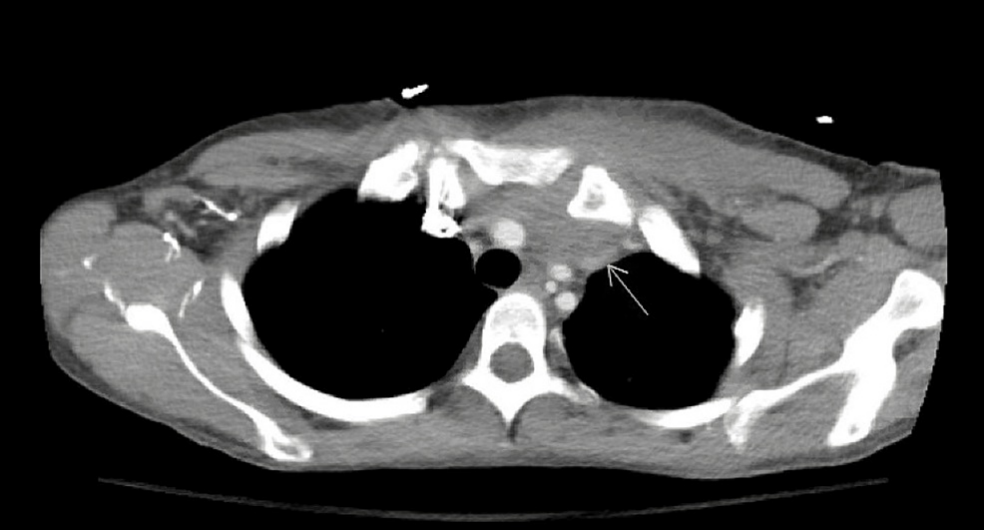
Contrast-enhanced axial CT scan of the upper chest showing soft tissue swelling, SCJ space widening with enlargement or thickening of the pectoralis muscle, and subcutaneous fat stranding
